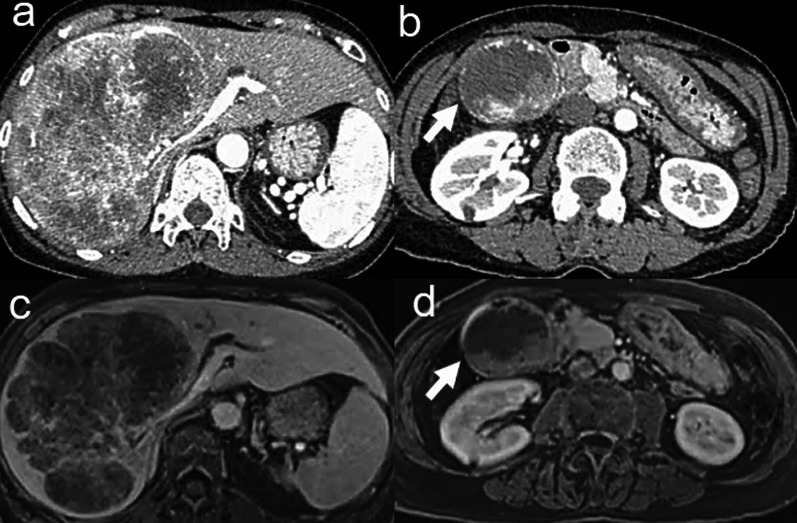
a Dynamic computed tomography (CT) shows a tumor measuring 12 × 7 cm in diameter in the right lobe of the liver. b A well-enhanced tumor is found in the gallbladder. c Contrast-enhanced magnetic resonance imaging (MRI) demonstrated the liver tumor. d The gallbladder tumor is also detected as an enhanced mass arrow
